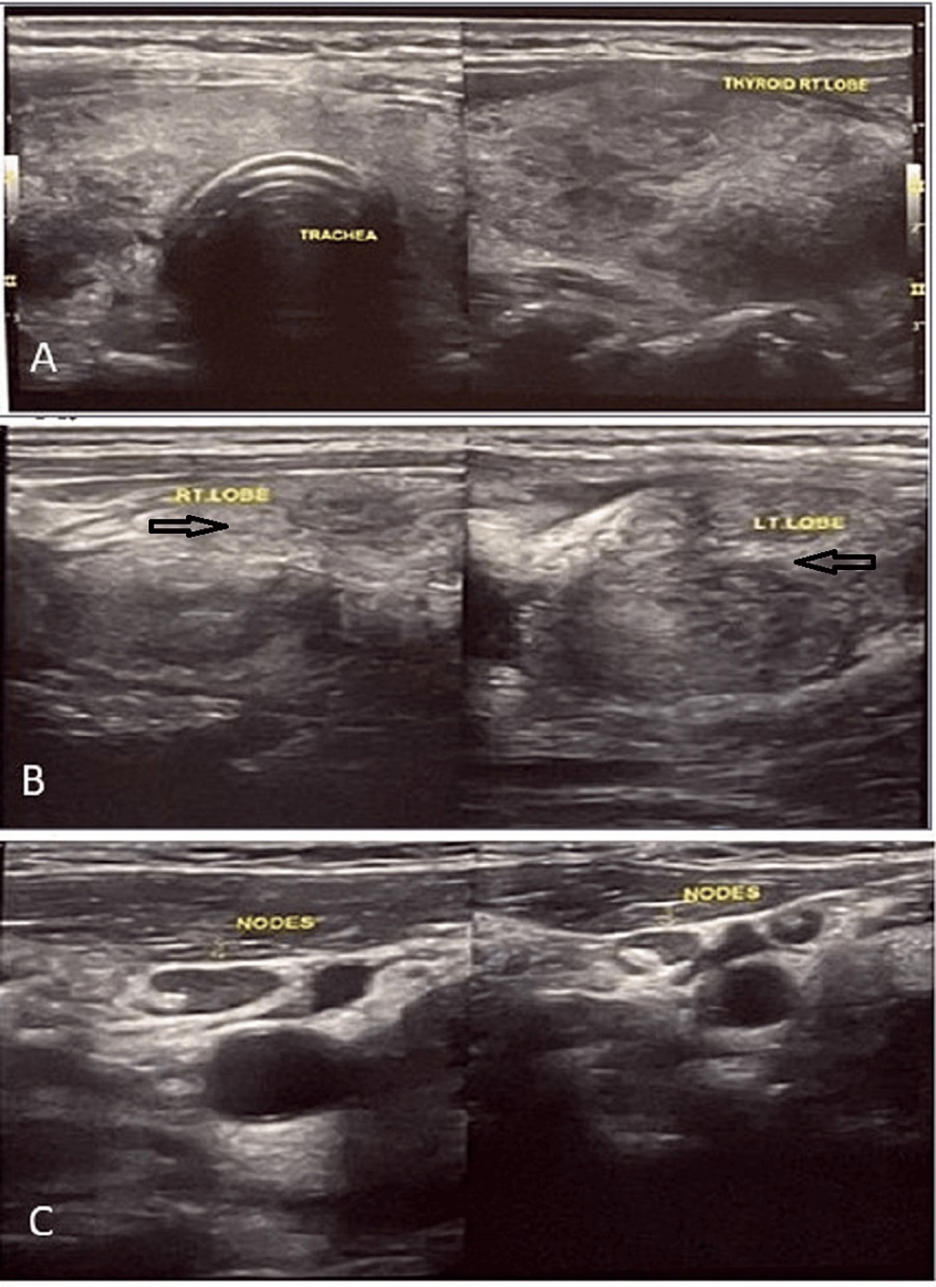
Ultrasonographic images of thyroid lobes. A and B: Enlarged isthmus, right and left lobe of the thyroid gland with coarse heterogeneous echo texture. C. Enlarged cervical lymph nodes with maintained fatty hilum.
